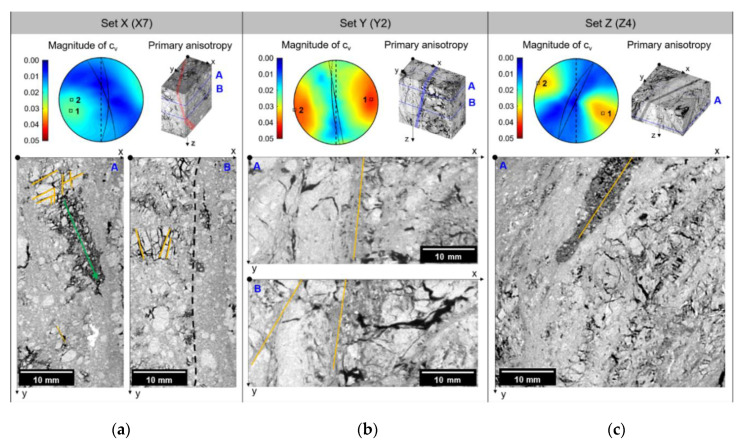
Stereonet plots of the coefficient of variation ($c_{v}$) computed by SPM and the used X-ray CT images. The orientation of the primary anisotropy is represented by a colored band in the 3D X-ray CT domain. The cross-sectional 2D images at depth A and B were obtained from the 3D X-ray CT domain. (a) Set X (X7). The yellow lines indicate the orientation of cracks in shattered grains. Green line shows the direction of trail suggesting the shear movements in the fault gouge. (b) Set Y (Y2). The yellow line shows the orientation of the narrow dark zone of matrix. This zone penetrates through the 3D domain in the z-direction. (c) Set Z (Z4). The yellow line indicates the orientation of the layered structure of fault gouge.
